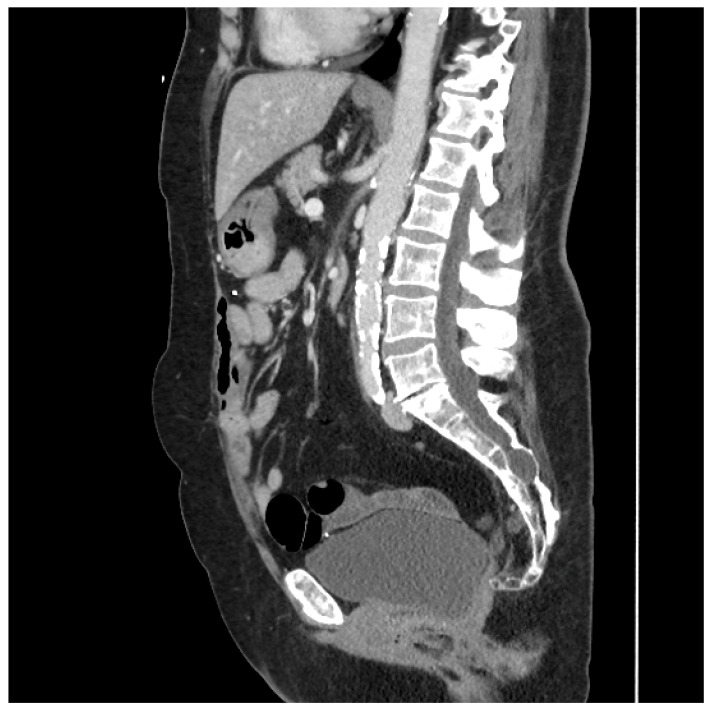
Sagittal CT scan performed four years after proctocolectomy and sigmoid mesocolic flap. The mesocolic flap is seen here filling the pelvis and adherent to the back of the bladder, forming a sling-like configuration, thus preventing the small bowel from adhering to the proctectomy resection bed in the pelvis. (Used with permission from the Mayo Foundation for Medical Education and Research; all rights reserved).
